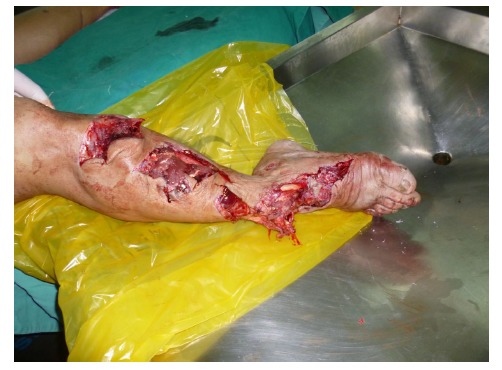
Extensive soft-tissue defects of the right lower leg and foot before debridement, accompanied by comminuted fracture of the proximal tibia, spiral fracture of the proximal fibula, fracture of the talus, and dislocation of the ankle joint.(a:clinical photo, b:x-ray of the leg, c: x-ray of the ankle).
